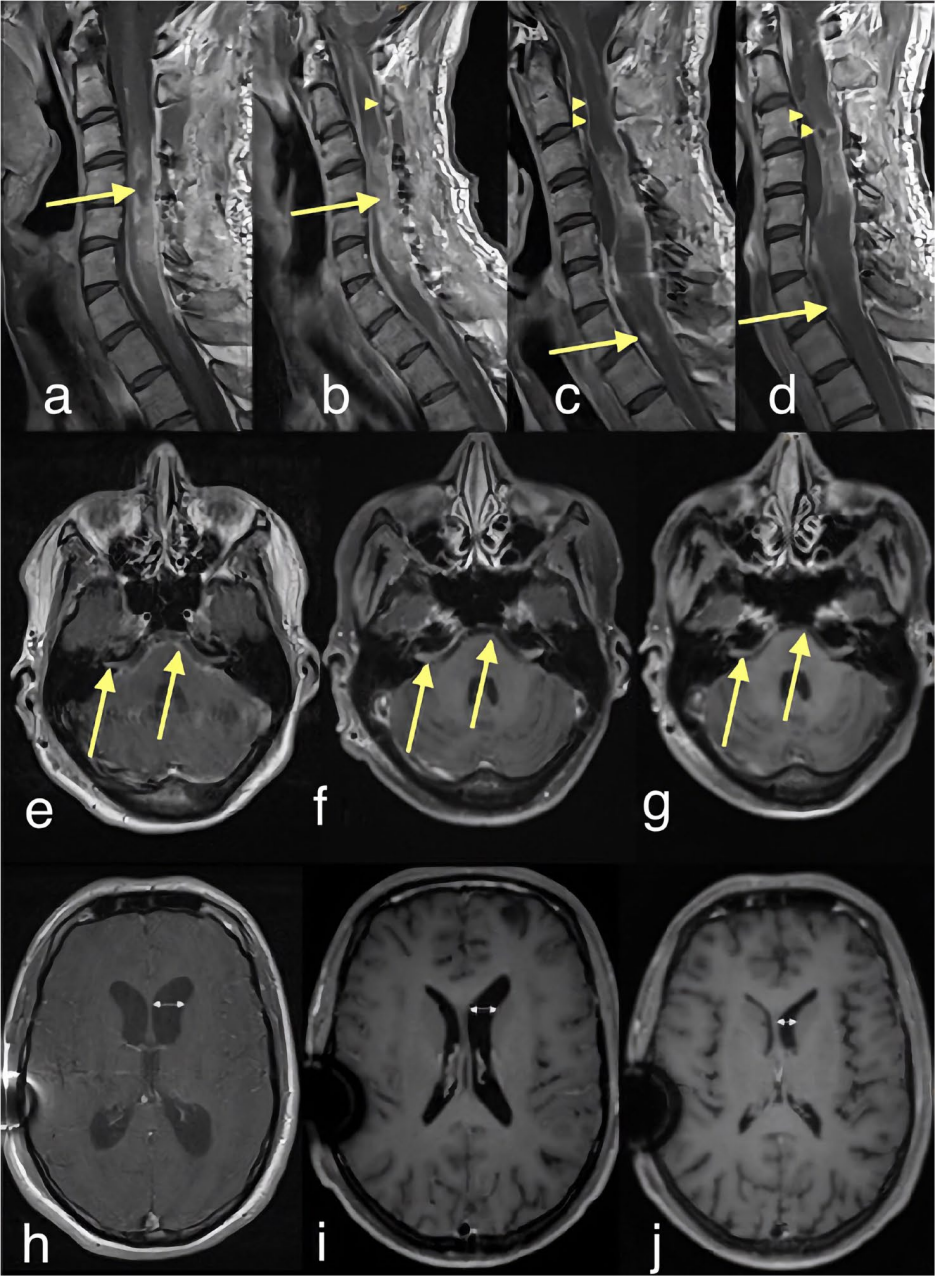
Contrast-enhanced T1w magnetic resonance imaging (MRI) of the cervical myelon (a-d) and neurocranium (e-j). Row a-d show the course of spinal involvement: b depicts tumour progression with leptomeningeal (arrowhead) and parenchymal involvement (arrow) within 18 days. Subsequently, the therapy was initiated. c and d show regression of contrast enhancement within 15 days (c) and after 25 days (d). e-g show regression of intracranial involvement with leptomeningeal contrast enhancement of the pons and cranial nerves VII and VIII. h-j demonstrate decrease in ventricular width after therapy initiation and shunt implantation
